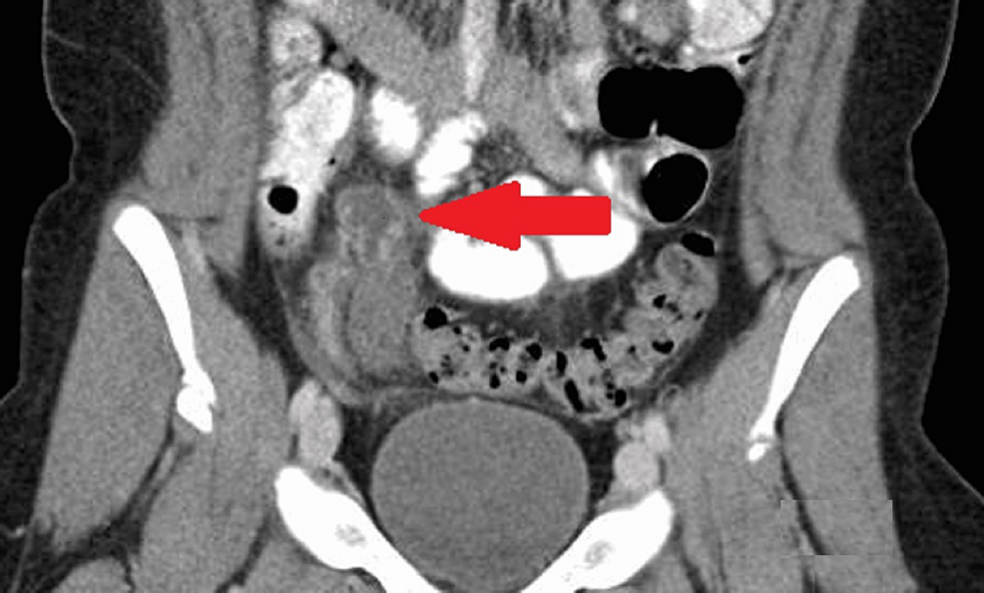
CT abdomen showing right hydrosalpinx
